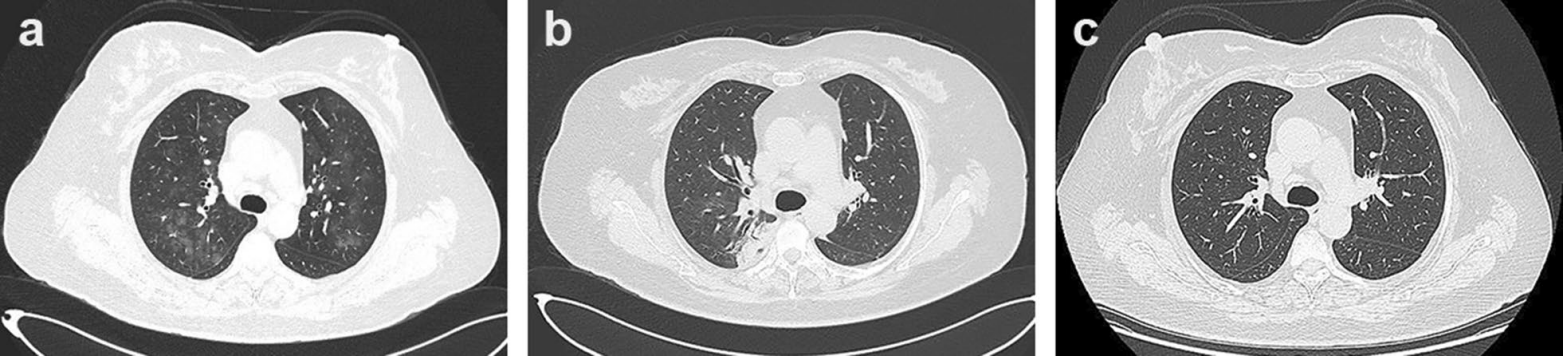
Chest CT images. a Preoperative chest CT image. b Chest CT image 1 week after surgery. c Chest CT image 2 months after surgery
